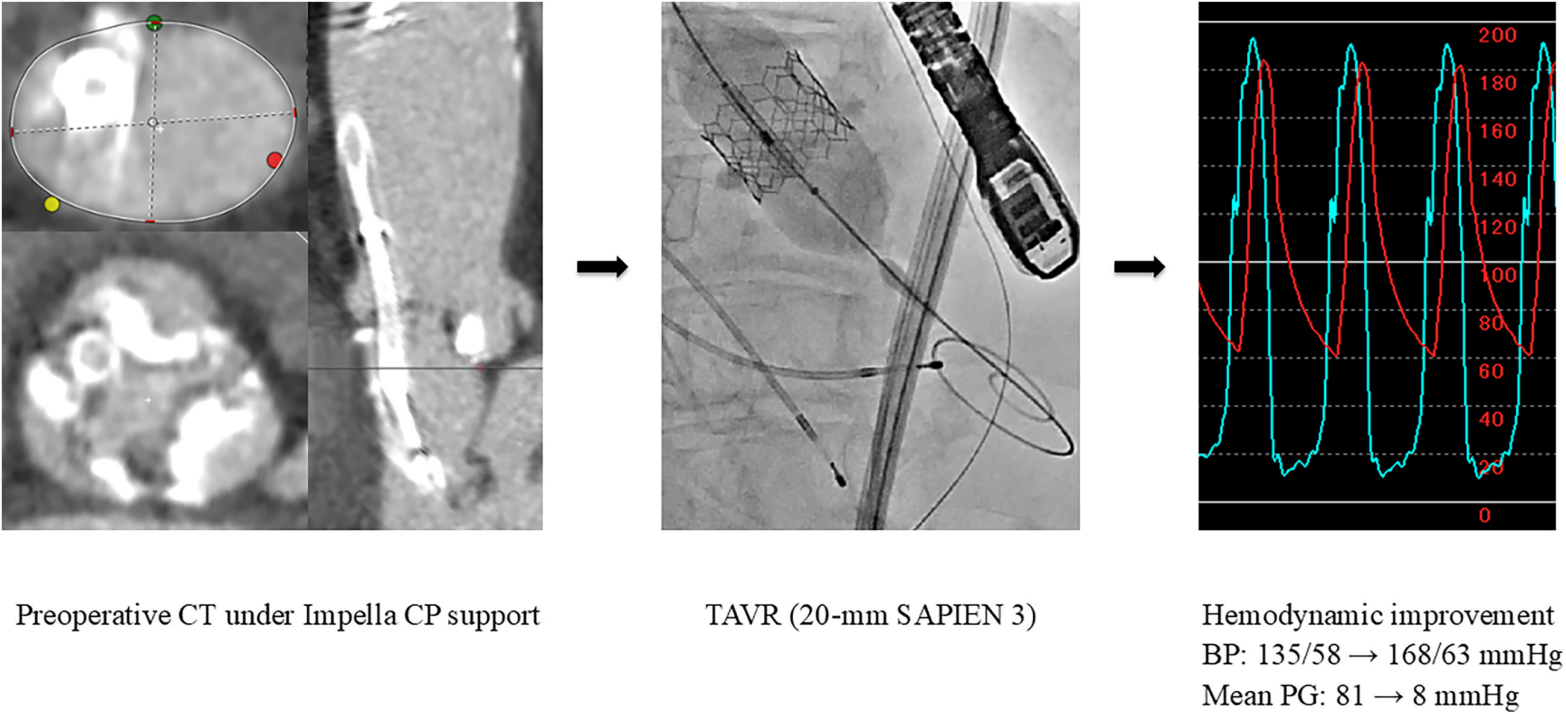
Preoperative CT imaging and urgent TAVR (Case 3). BP, blood pressure; CT, computed tomography; PG, pressure gradient; TAVR, transcatheter aortic valve replacement.
